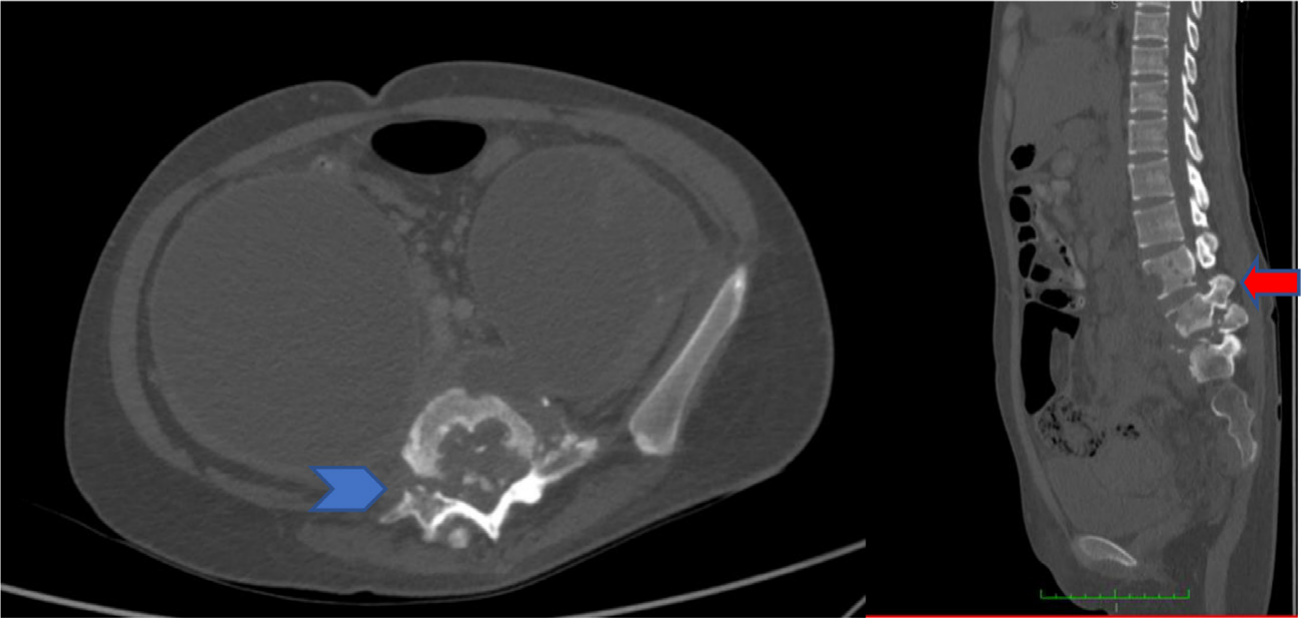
Abdominopelvic CT with bone window: (A) axial plane showed destruction of the L5 vertebra into the spinal cord with abscess formation in the prevertebral and paravertebral spaces, psoas abscess, and retro vertebral space (blue arrow); (B) the sagittal bone window showed bone destruction of the L4–5 vertebrae and S1 (red arrow)
